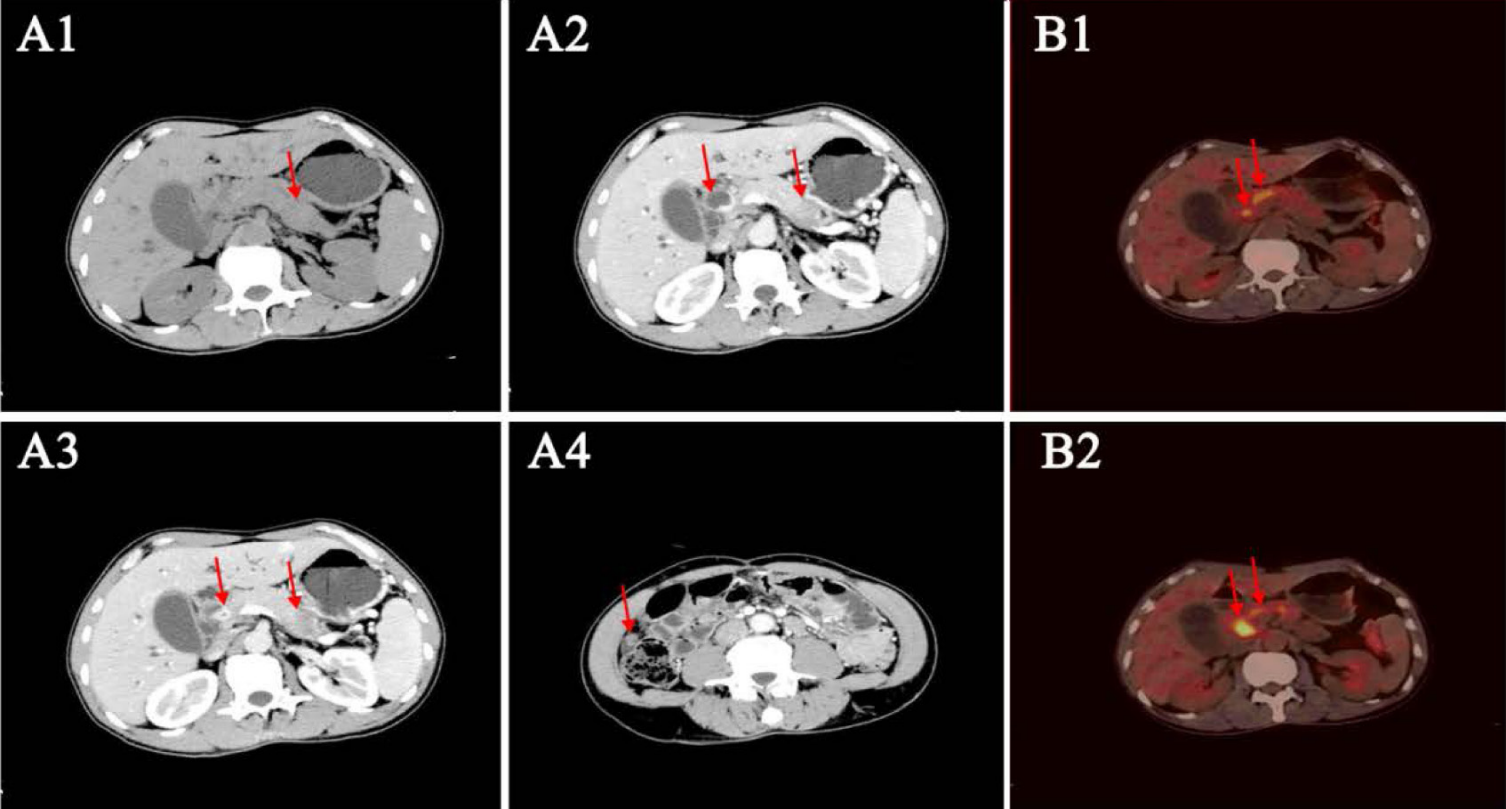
Radiological assessments of the patient (A1) Unenhanced computed tomography (CT) depicts an equal-density mass in the tail of the pancreas; the common bile duct is not clear. (A2–A3). Contrast-enhanced CT shows thickening of the wall of the common bile duct with significantly persistent enhancement. The mass in the pancreas tail is of low density compared with the dramatically enhanced mass in the pancreas. (A4). Contrast-enhanced CT shows mild enhancement of a mass in the right peritoneum. (B1–B2). Positron emission tomography (PET)/CT assessment of the patient. The PET/CT shows hypermetabolic masses in the comment bile duct and the head of the pancreas.
